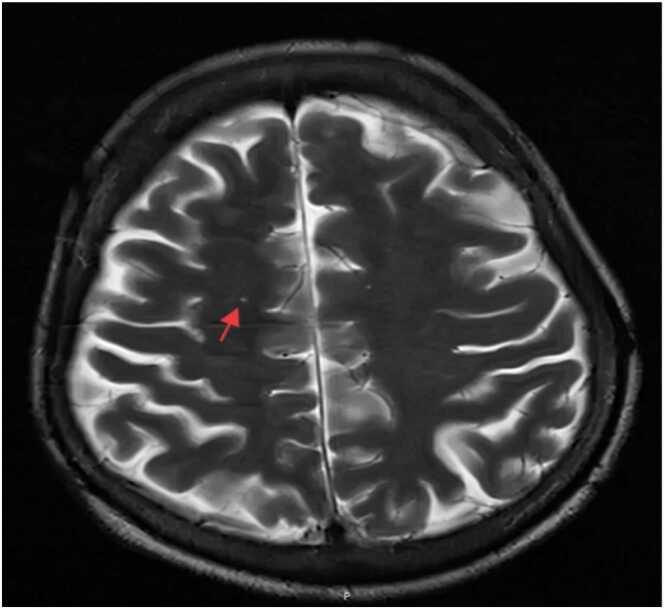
Axial brain MRI (FLAIR sequence): Periventricular white matter ischemia (arrow); no acute cerebral infarction/hemorrhage.
